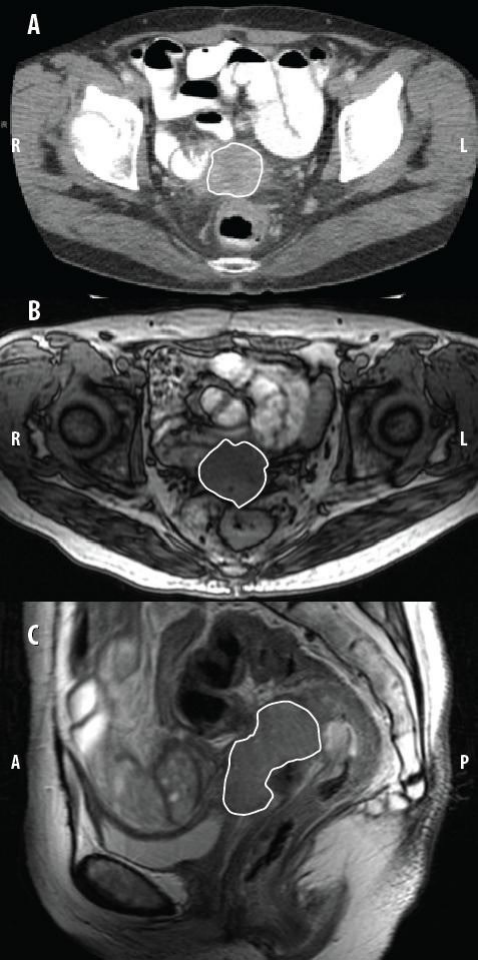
Diagnostic imaging: (a) axial CT image of the pelvis obtained at presentation shows a supravaginal 3.7×3.9cm primary peritoneal clear cell carcinoma (PP‐CCC) and an (b) axial and (c) sagittal T2‐weighted MR image of the pelvis obtained one month following presentation which shows a supravaginal 5.6×3.7×3.5cm primary peritoneal clear cell carcinoma. The lesion appears inseparable from the anterior wall of the proximal rectum.
